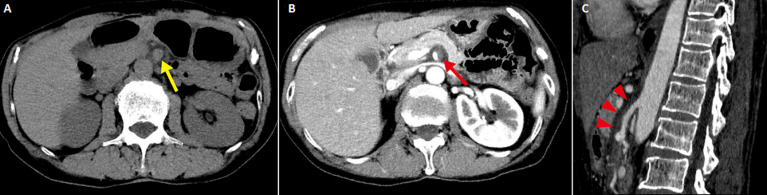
A) non-contrast-enhanced computed tomography image of the abdomen revealing the dilation of the superior mesenteric artery (yellow arrow); B, C) contrast-enhanced computed tomography image of the abdomen depicting the dissection of the superior mesenteric artery (red arrow and arrowheads)
